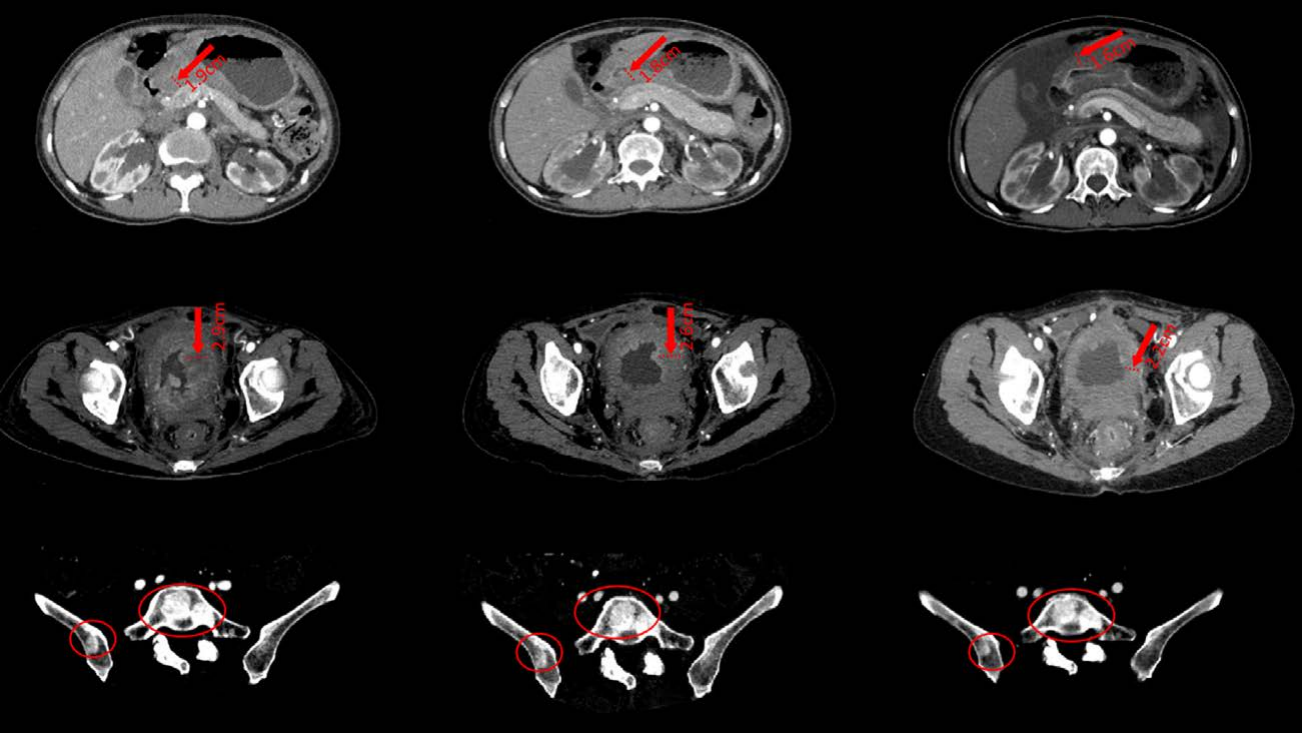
Changes in lesion seen on CT scan. CT = computed tomography.
